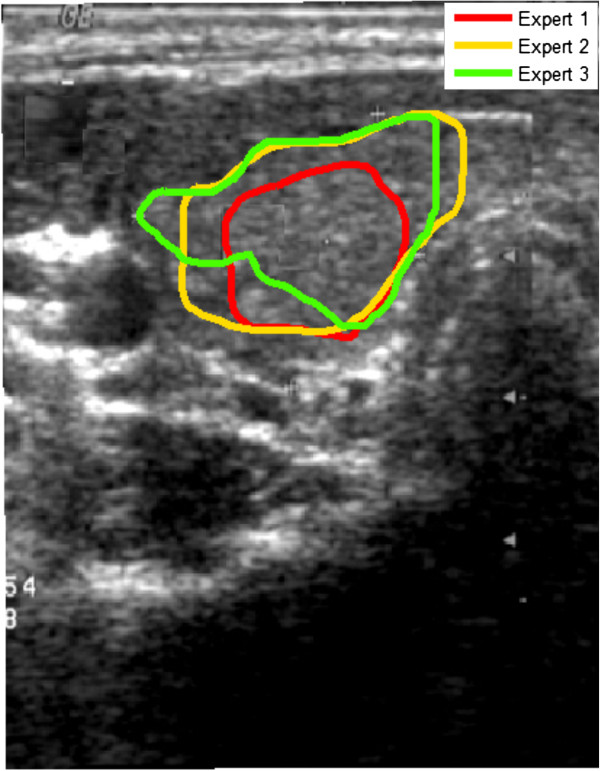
Ultrasound image of the cross section of the thyroid. The thyroid lobe areas marked by 3 experts are highlighted in colours. The visible differences are due to different approaches to the thyroid lobe, an attempt to eliminate shadows and other artefacts. According to some experts, shadows and other artefacts are characteristic of the thyroid lobe and should not be eliminated from the calculation.
